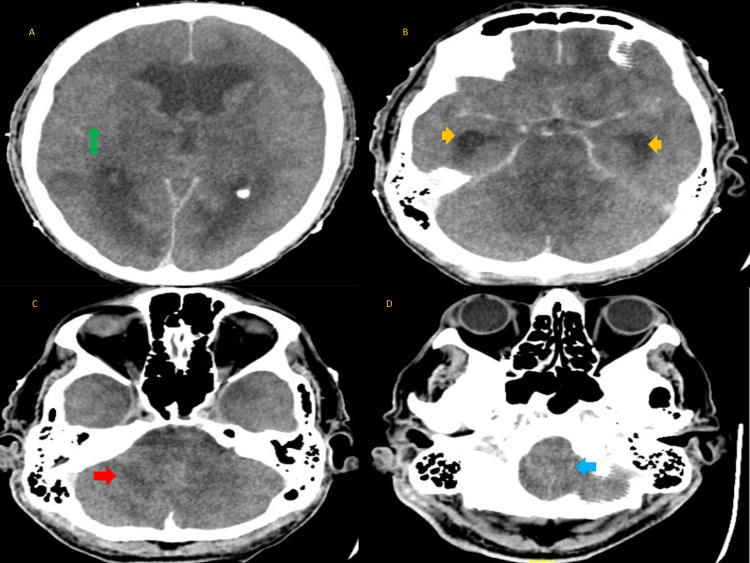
CT head with contrast Anoxic injury:  (A) diffuse cerebral edema loss of sulci and gyri (green arrow), (B) hydrocephalus (yellow arrows), (C)  cerebellar edema (red arrow), and (D) herniation (blue arrow).
